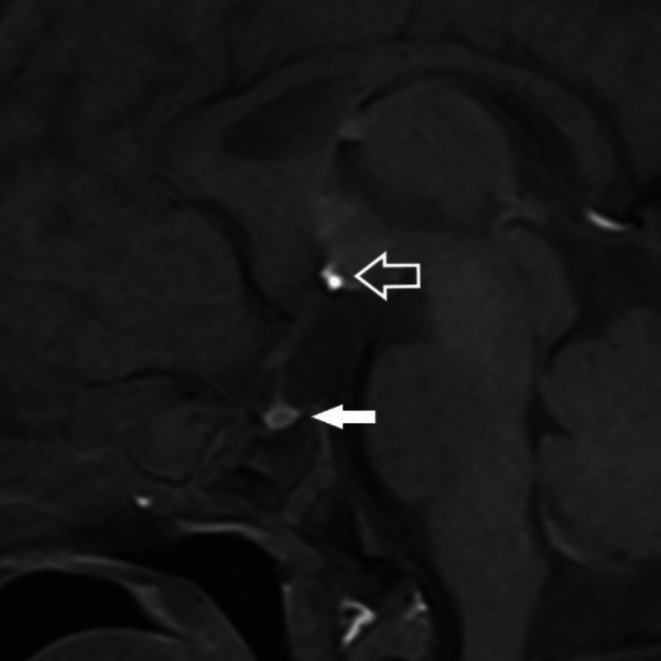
Sagittal T1‐weighted MR image of the pituitary gland shows the adenohypophysis situated in the pituitary fossa (solid arrow). The neurohypophysis is not situated in the pituitary fossa but ectopically situated in the floor of the third ventricle‐hypothalamus (open arrow).
